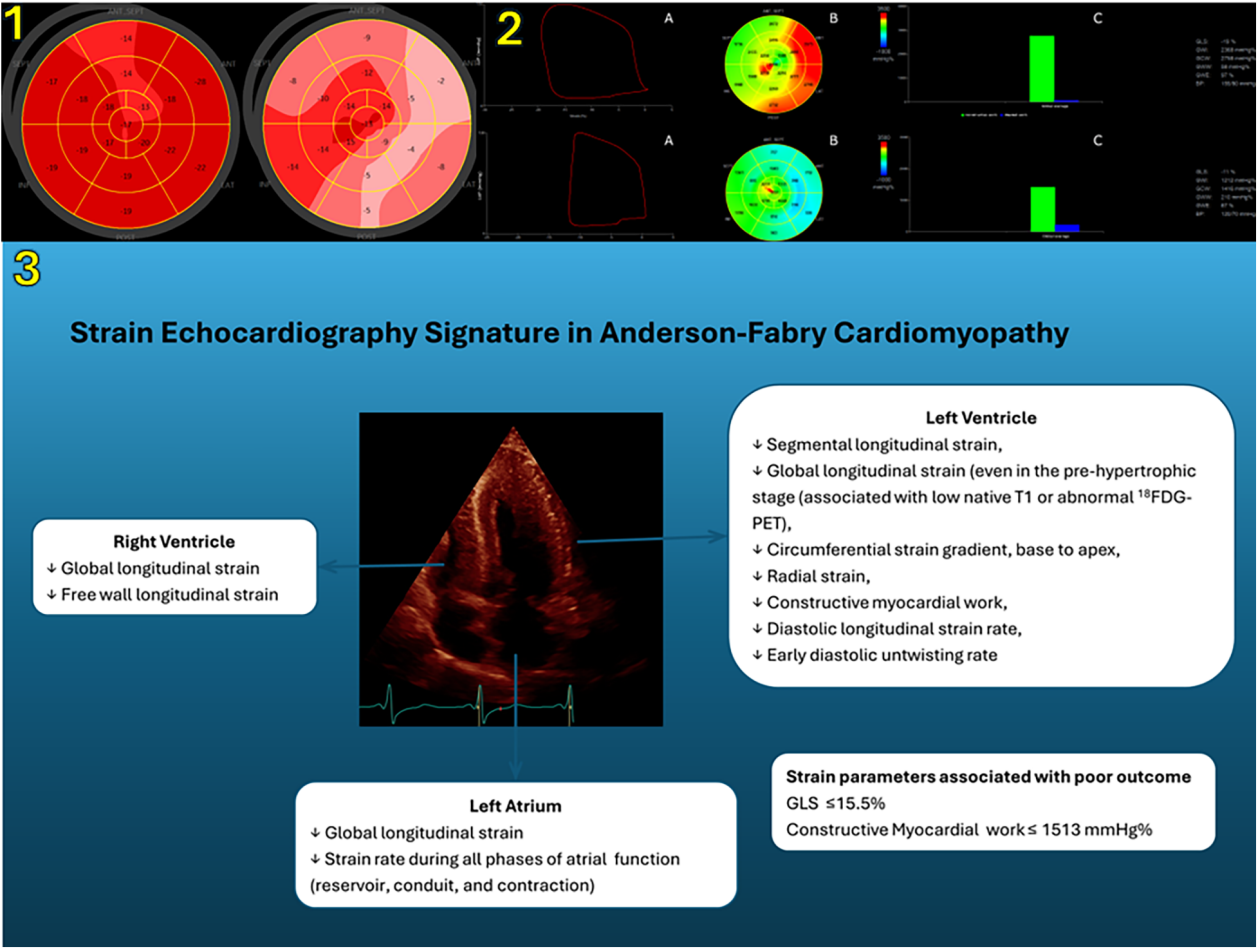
Panel 1. Left ventricular longitudinal strain measurements by means of two-dimensional speckle tracking echocardiography. Bull’s-eye rendering of LV longitudinal strain from a 42-year-old female AFD patient without LV hypertrophy (left) and a 53-year-old male AFD patient with LV hypertrophy (right). Panel 2. (A) Representative example of pressure–strain loops by estimated LV pressure and LV longitudinal strain; (B) Bull’s eye plot showing segmental LV myocardial work index in a 17-segment model; (C) constructive work (green column) and wasted work (blue column) values from the same patients as in the panel 1 (*upper*, female patient without LV hypertrophy; *lower*, male patient with LV hypertrophy). Panel 3 shows the role of strain echocardiography in the characterization of cardiac involvement in AFD. LV, left ventricular; AFD, Anderson–Fabry disease; SEPT, septal; LAT, lateral; ANT, anterior; INF, inferior; POST, posterior; LVP, left ventricular pressure; GLS, global longitudinal strain; GWI, global work index; GCW, global constructive work; GWW, global wasted work; GWE, global work efficiency; BP, blood pressure; ^18^F-FDG PET, ^18^F-luorodeoxyglucose uptake by cardiac positron emission tomography.
